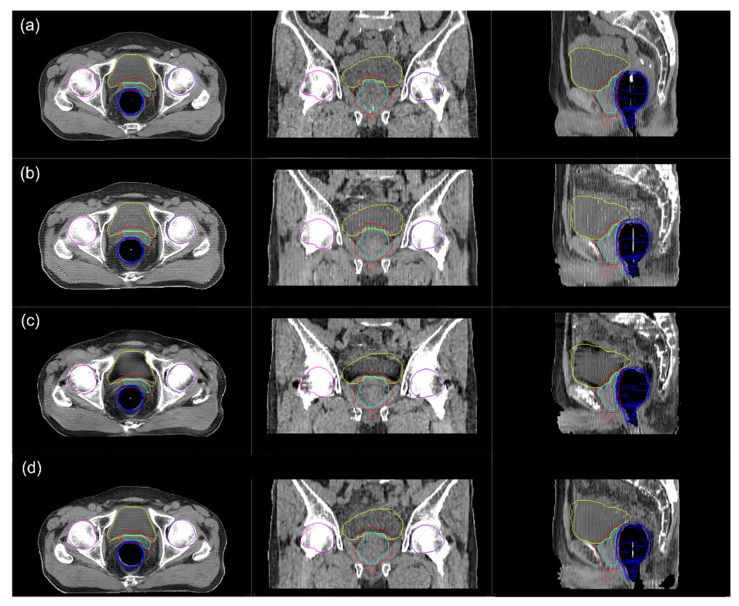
Example of original (a) and synthetic computed tomography generated from generative and adversarial network (GAN) (b), cyclo-consistent GAN (c) and reference-guided cyclo-consistent GAN (d) (left; axial view, middle; coronal view; right sagittal view).
